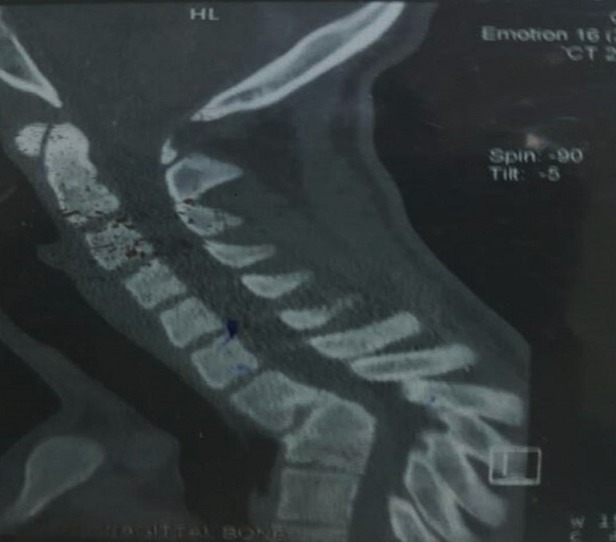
Preoperative CT Imaging with spinal cord compression at level T1/T2
